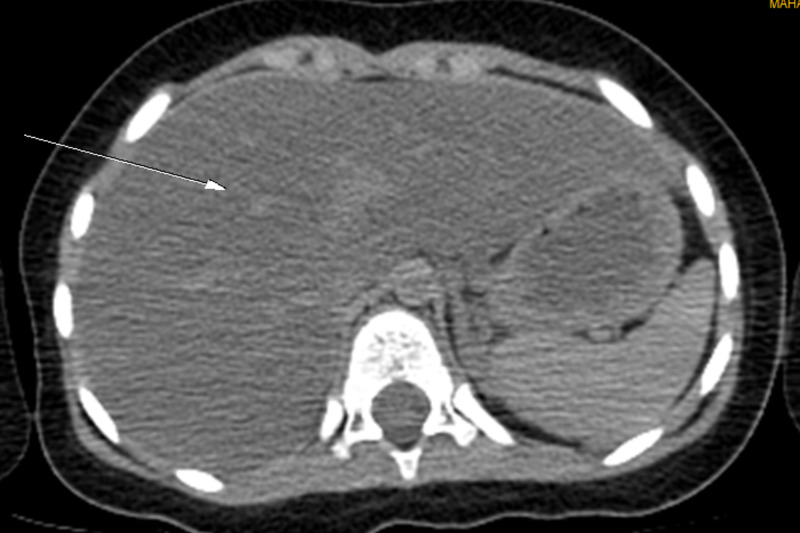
Abdominal CT scan section showing mild hepatic steatosis (arrow)
